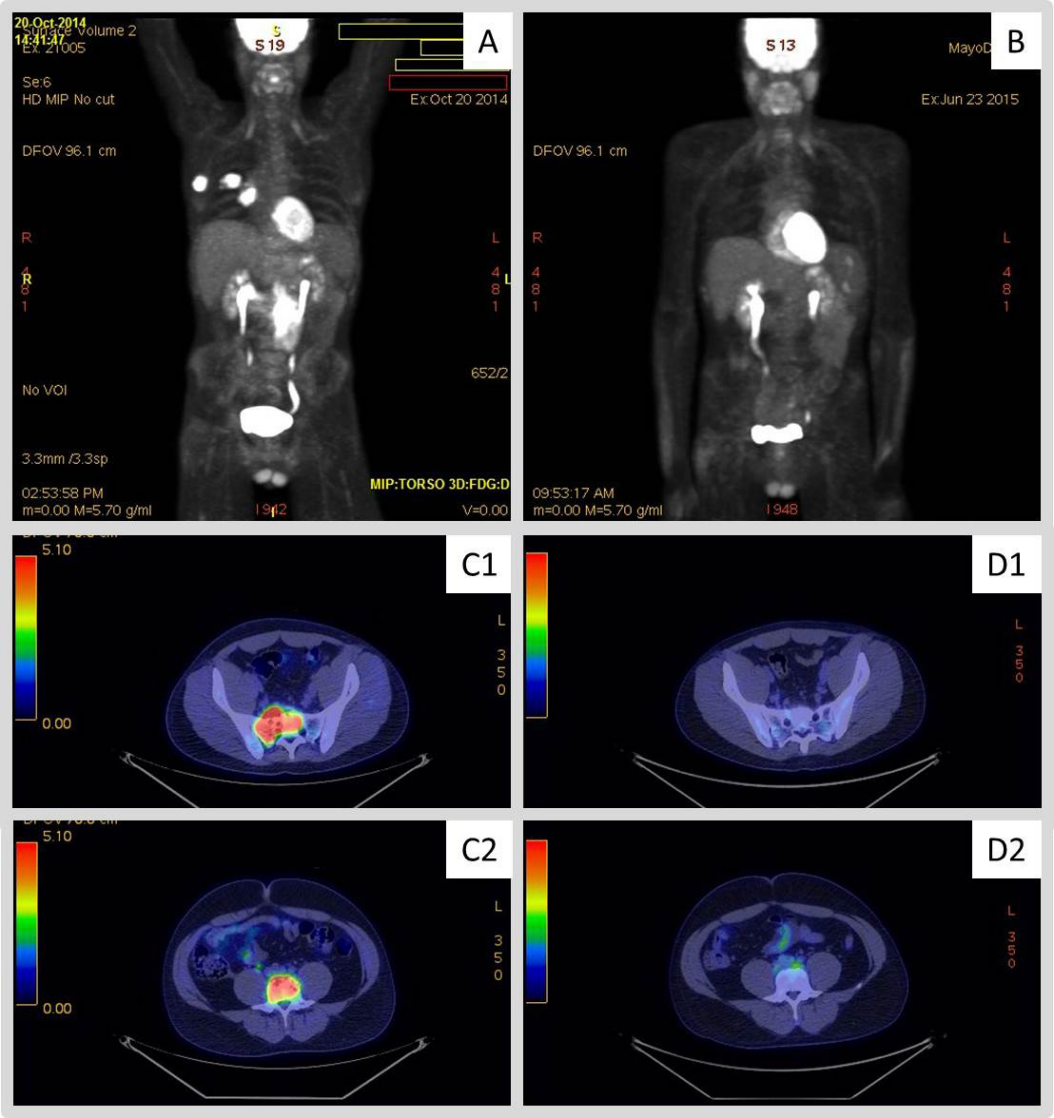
18F-fluorodeoxy-glucose–positron-emission tomography (FDG-PET) images obtained to evaluate response to therapy with pembrolizumab Panels A. and (C1/C2) display pre-treatment scans for patient 1 and 2, respectively. Hypermetabolic masses are observed in the abdomen, lateral right chest wall soft tissue extending into the lung parenchyma, and right hilum on panel (A). Panel (C1) displays hypermetabolic skeletal lesion involving upper right sacrum while (C2) demonstrates involvement of L4. Panels (B) and (D1/D2) display follow-up scans obtained to evaluate response to therapy in patient 1 and 2, respectively. Panel B. displays PET-CT scan obtained on patient 1 after 3 cycles of pembrolizumab showing no evidence of FDG-avid malignancy. Panel (D1 and D2) displays PET-CT scan obtained on patient 2 after 4 cycles of pembrolizumab showing resolution of FDG-avid bone lesions.
